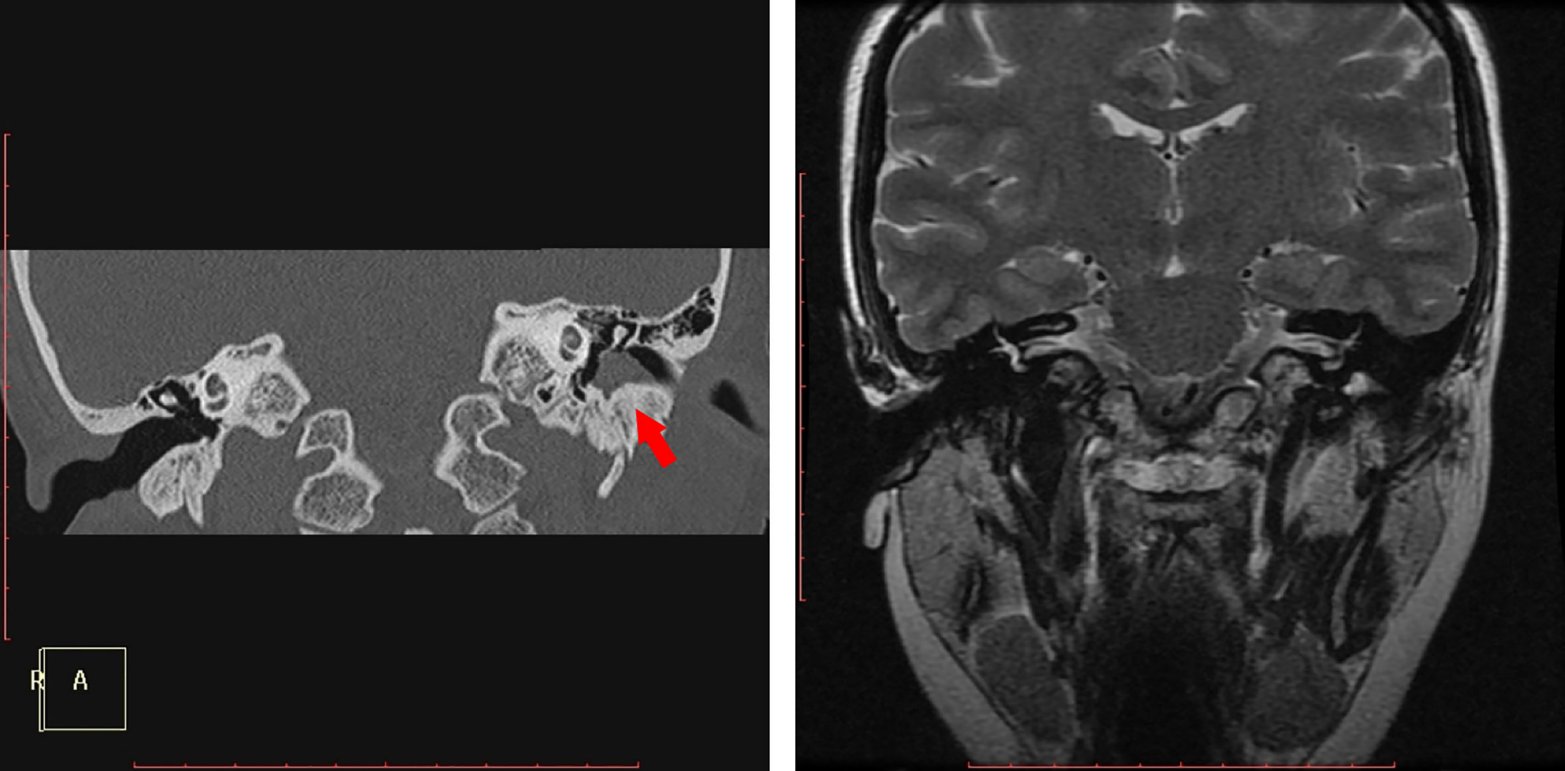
(case 2): Ceruminous gland adenoma in the bony portion of the left external auditory canal. Coronal CT showed a soft mass, the tympanic bone was mildly involved and was highlighted by a red arrow(a). Coronal T2WI showed a high signal intensity in the deep part of the EAC.
